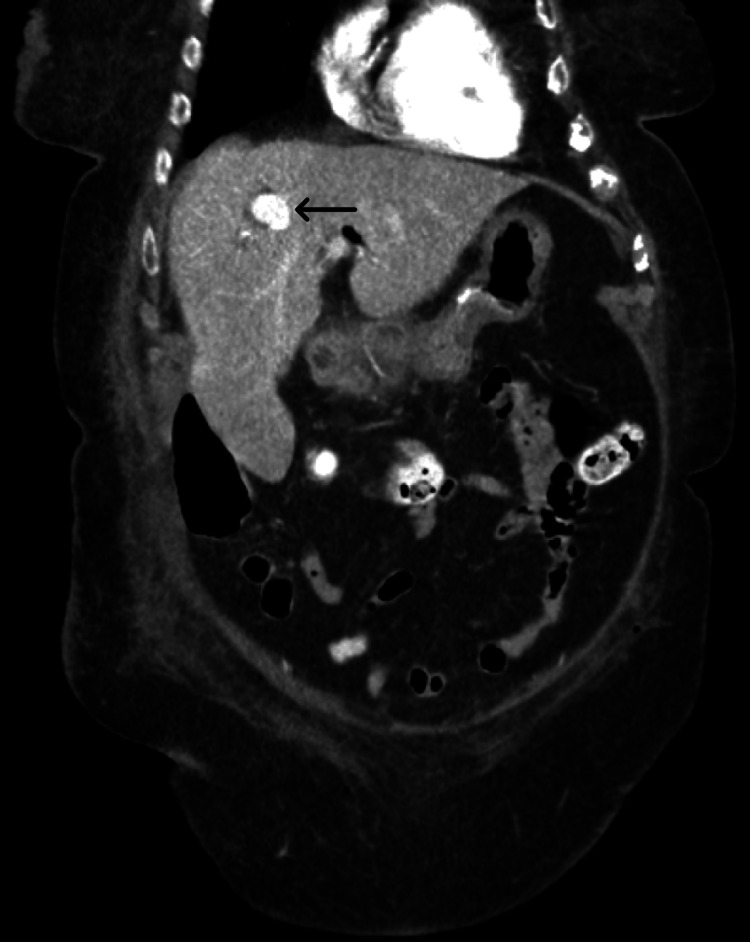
Coronal CT image showing a 1.8-cm intrahepatic pseudoaneurysm (arrow) in the right hepatic lobe.
